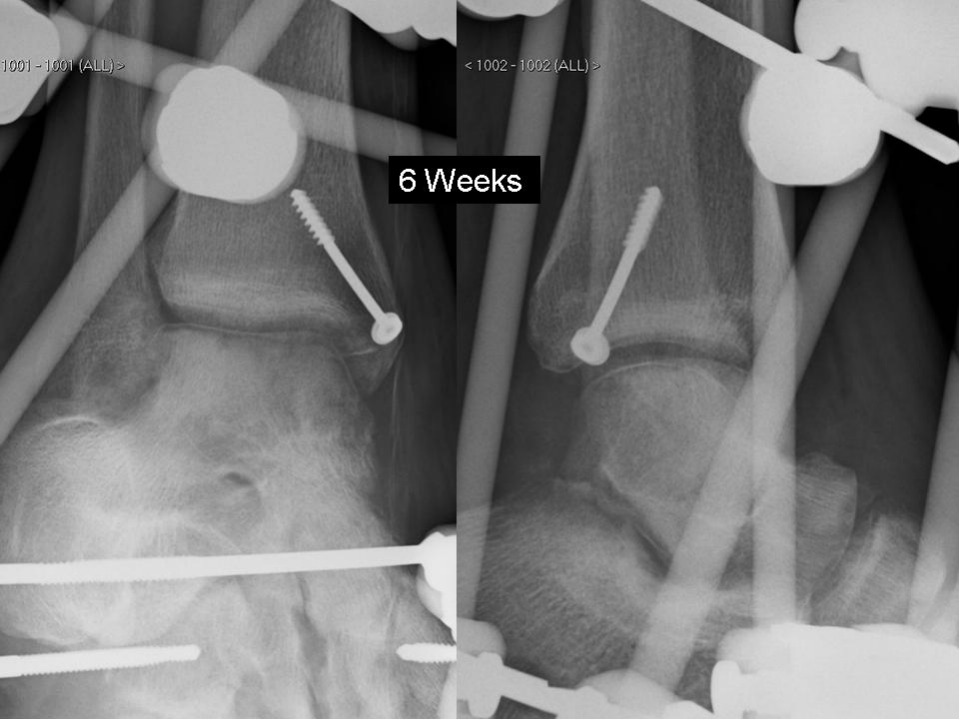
Anterio-posterior and lateral radiograph at 6 weeks.
